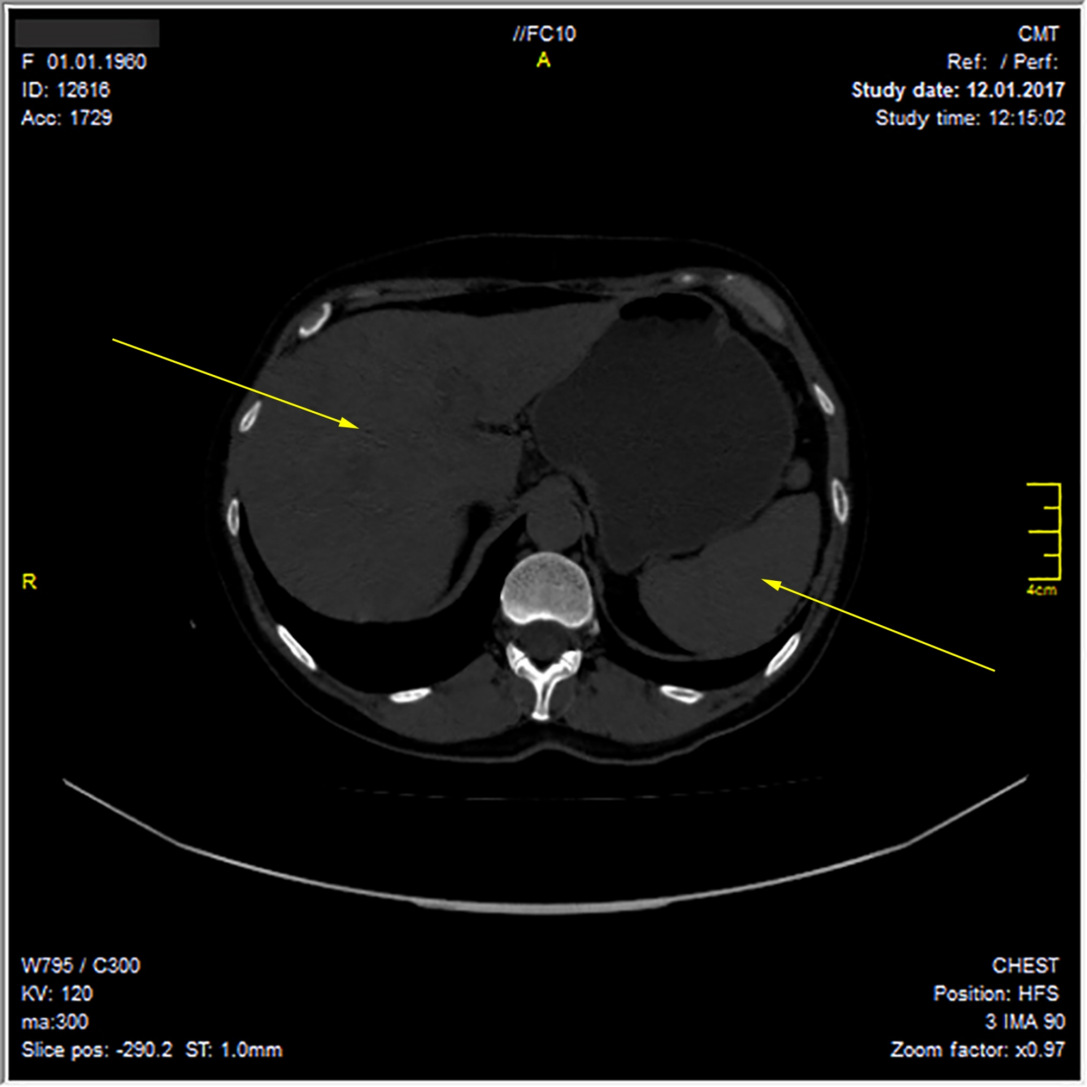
Hepatomegaly (craniocaudal length of the liver was 170 mm), splenomegaly (111x49 mm) according to the computed tomography of the abdominal cavity
